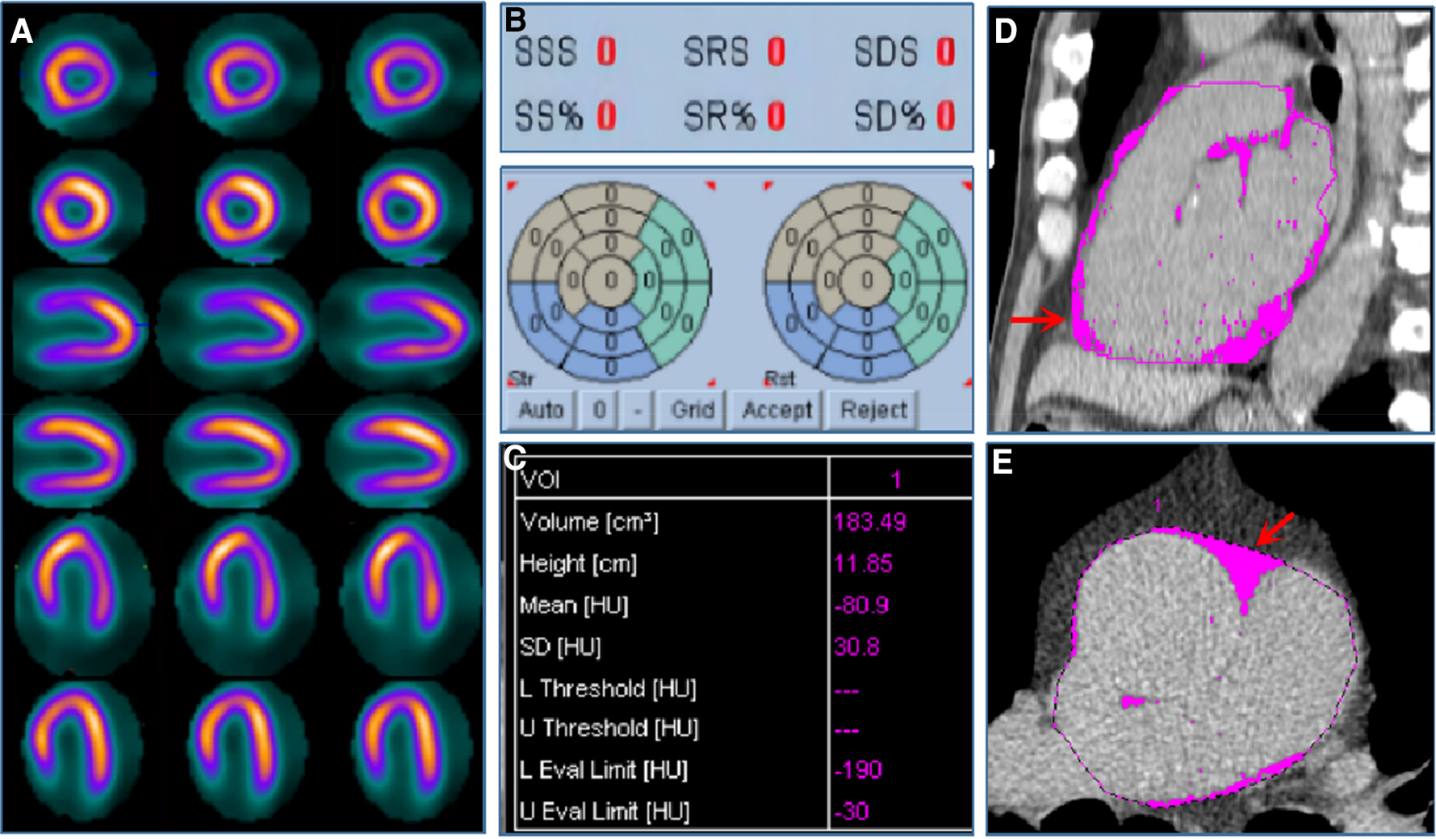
Case example. A 57-year-old male patient was admitted to the hospital with atypical chest pain for half a month. The patient was diagnosed with suspected CAD and received stress–rest MPI. (A) shows stress (the odd row) and rest (the even row) MPI of the short axis, vertical long axis, and horizontal long axis images, respectively, indicating the normal MPI with SDS equaled to 0 (B). (C) shows EFV was 183.49 cm^3^. (D,E) show EAT in pink of coronal and sagittal views from non-contrast CT scan.
